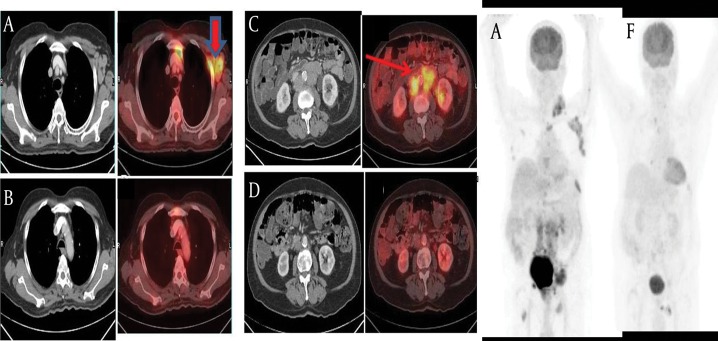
Transaxial CT and Fused FDG PET/CT Images Figure 2A shows multiple enlarged lymph nodes in the left cervical region, with the largest node measuring 2.6 cm in the short axis (SUVmax 6.0 gm/ mL). Figure 2C shows a conglomerate mass of the abdominal lymph nodes with the largest node measuring 6.8 cm in the short axis (SUVmax 5.9 gm/mL). There was complete morphological and metabolic disappearance (Figure 2B and 2D) of these nodes after androgen ablation and hormonal treatment. Maximum intensity projection (MIP) PET images show pretherapy disease burden (Figure 2E) and the dramatic response post-treatment (Figure 2F).
